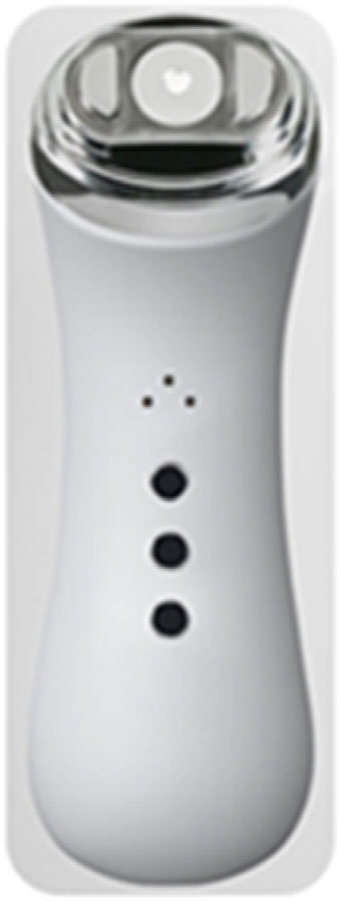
A novel high‐intensity focused ultrasound (HIFU) device
